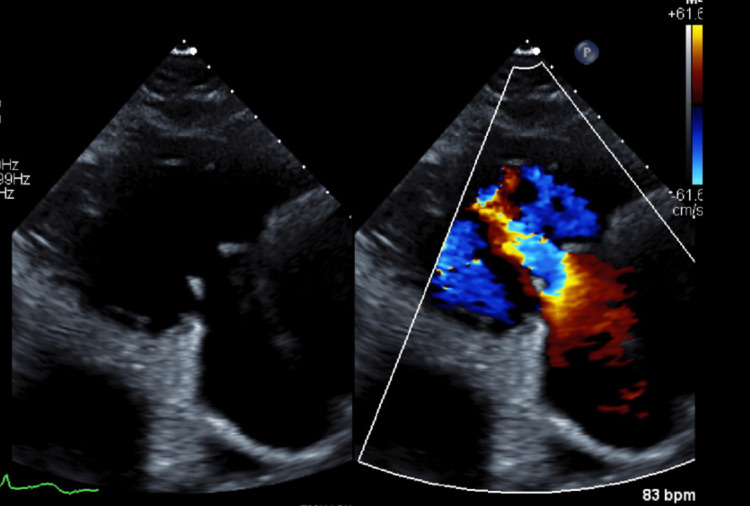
A color Doppler ultrasound of the tricuspid valve showed a jet of blood flowing downward (away from the transducer), indicating tricuspid regurgitation.
